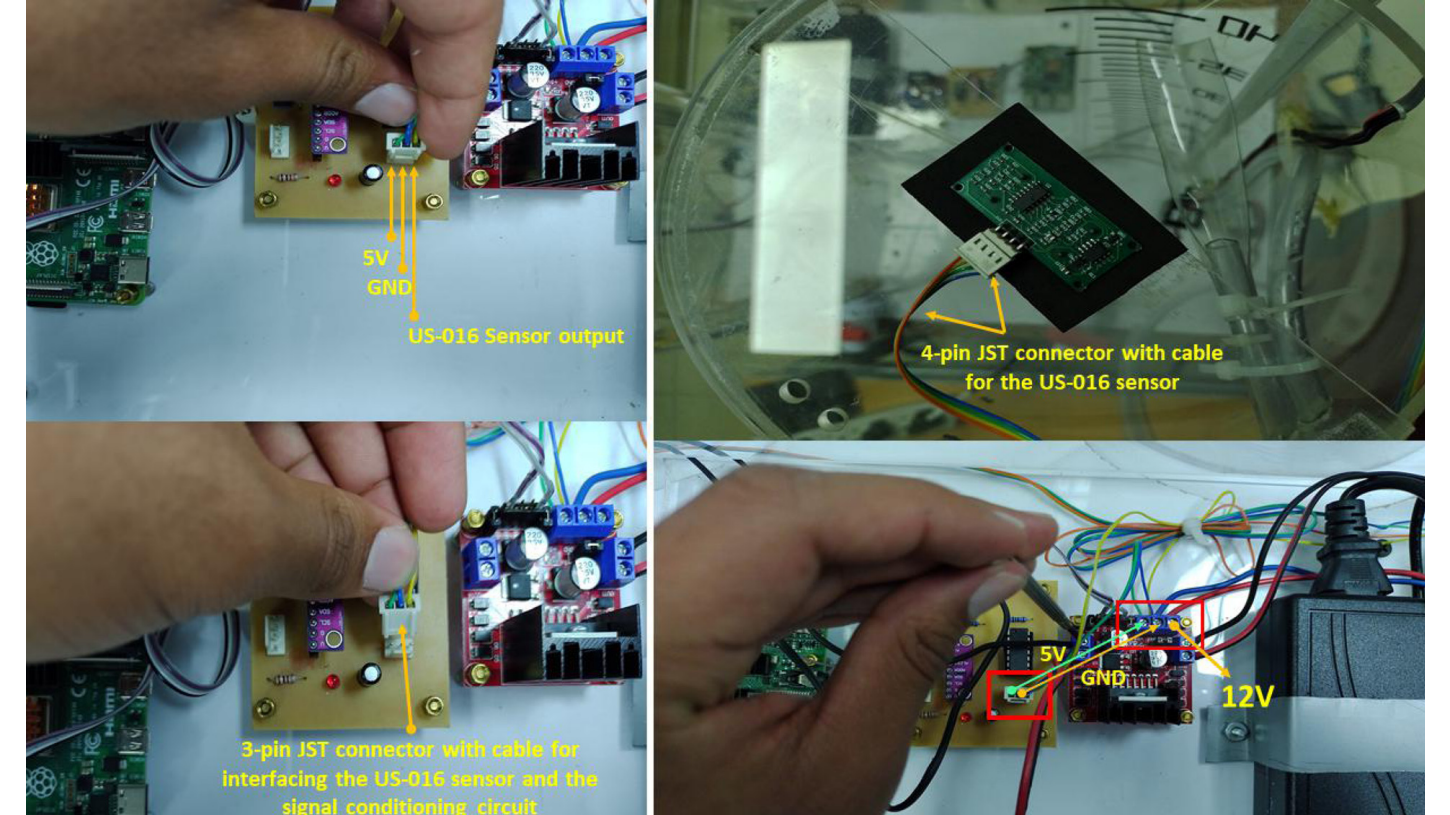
Wiring connections for the ultrasonic sensor and signal conditioning circuit.
